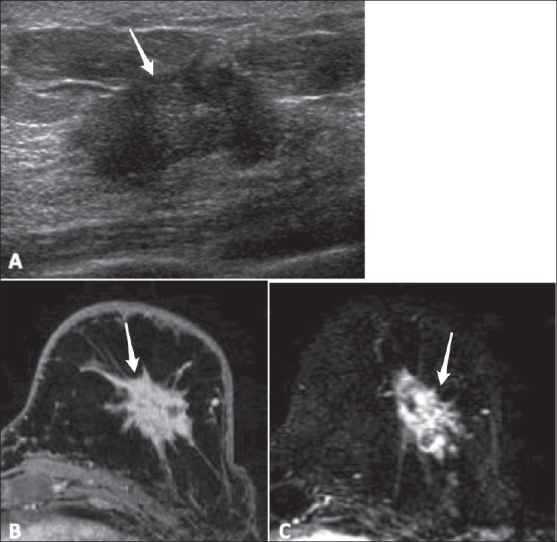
A 55-year-old woman with a palpable abnormality. USG (A) shows a hypoechoic, irregular mass (arrow) with angular margins, classified as BI-RADS 5. Biopsy of the mass showed stage II invasive ductal carcinoma. A pre-operative breast MRI with high-resolution delayed post-contrast (B) and subtracted (C) images, demonstrates a heterogeneously enhancing, irregular mass (arrows) with spiculated margins, classified as BI-RADS 6
